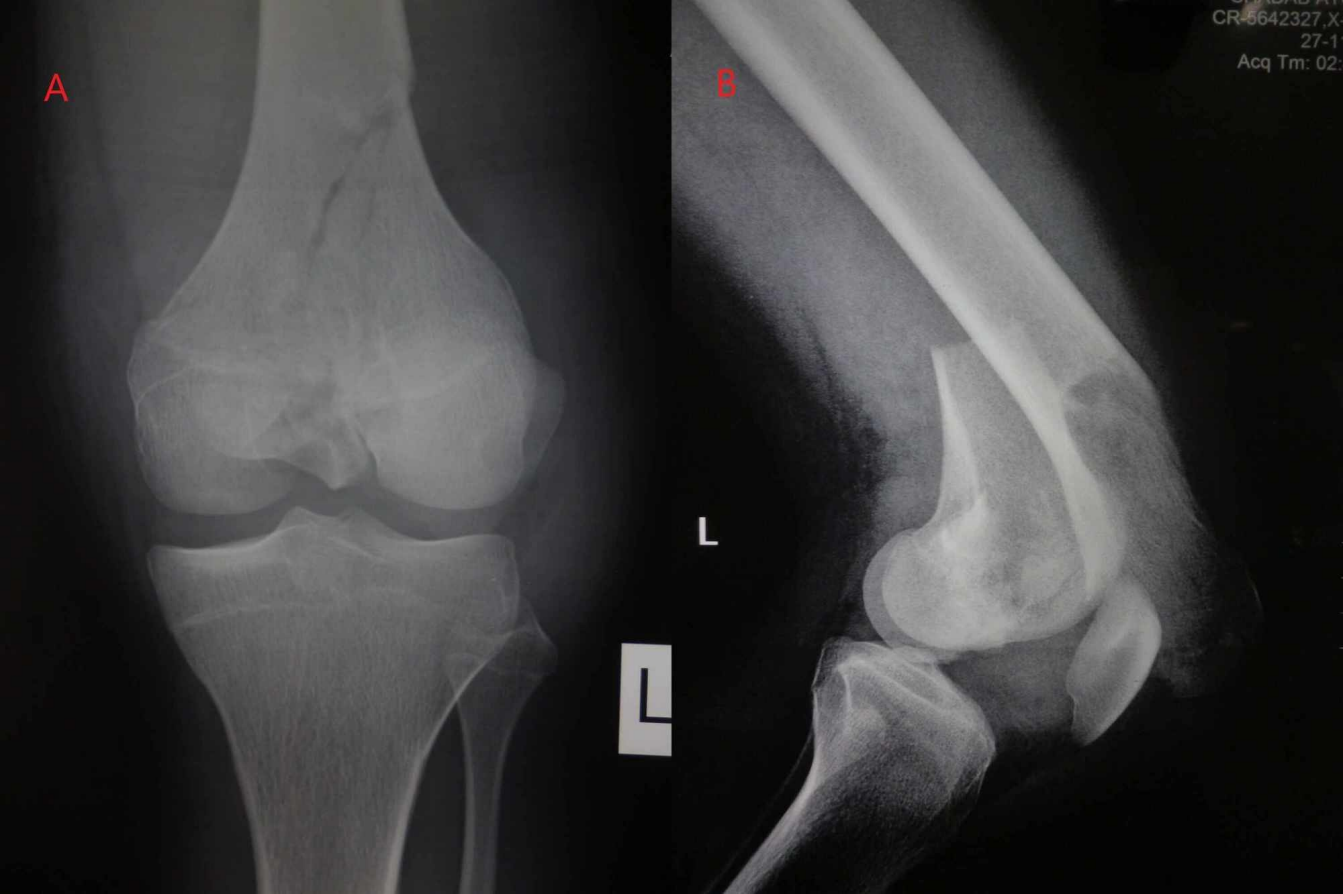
Preoperative radiographs of the knee A: anteroposterior view; B: lateral view. The images show distal femur fracture
